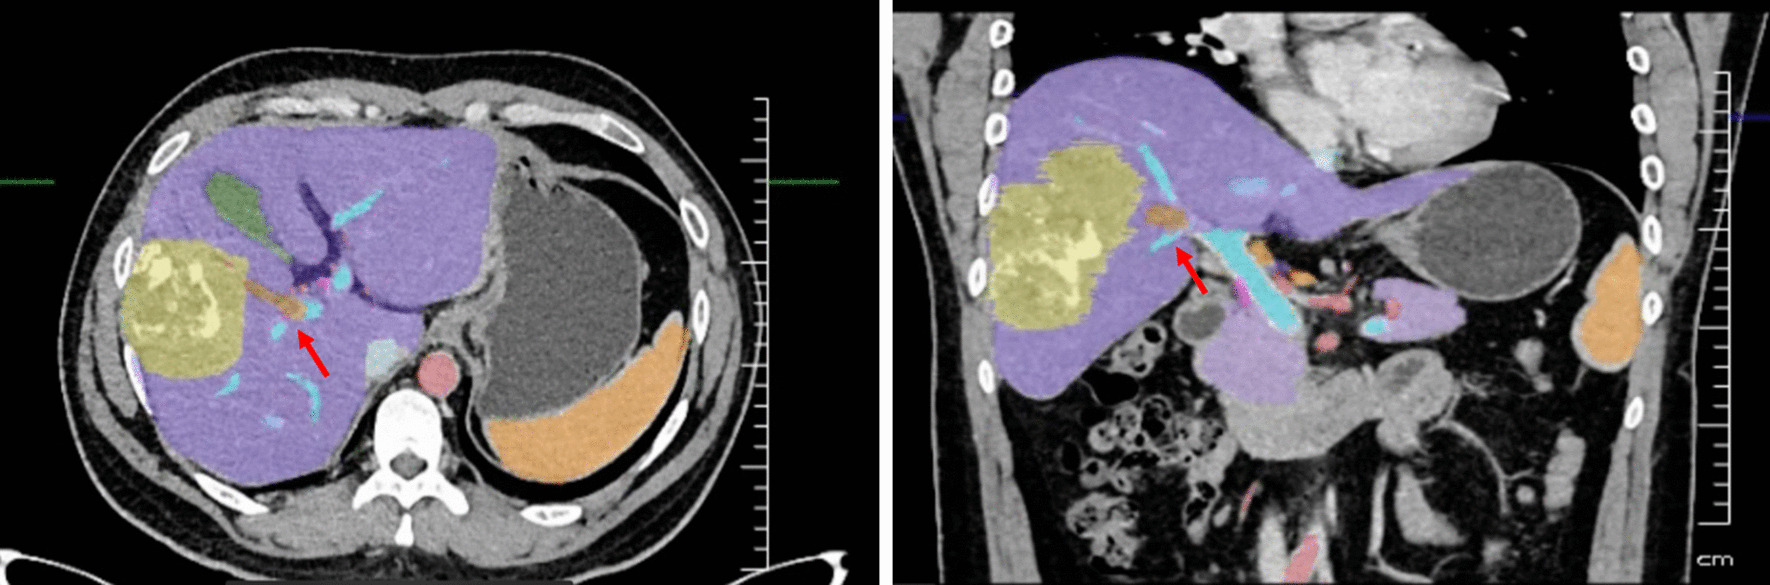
Preoperative Computed Tomography scan of liver, a right liver space-occupying lesion was found. The lesion was considered primary liver cancer with embolus of portal vein right anterior branch (red arrow)
